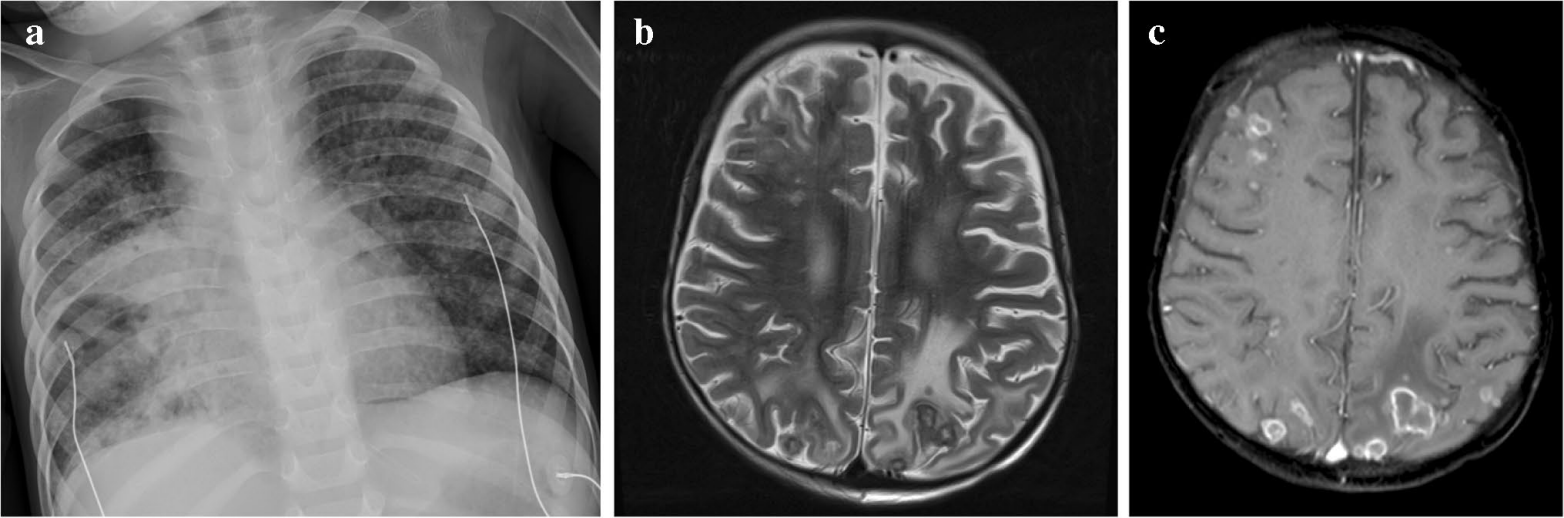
A 9-month-old boy presenting with a decreased level of consciousness. a Posteroanterior chest radiograph shows paratracheal and hilar lymphadenopathy and a diffuse micronodular pattern in keeping with miliary tuberculosis. b, c Axial T2 (b) and T1 post-gadolinium (c) magnetic resonance imaging  show innumerable lesions at the grey/white matter junction and meninges, some of which are hypointense (caseating tuberculomas) and some of which have T2 hyperintense centres (liquifying caseating tuberculomas) and show surrounding oedema (b). There is peripheral enhancement on the T1 post-gadolinium image (c)
